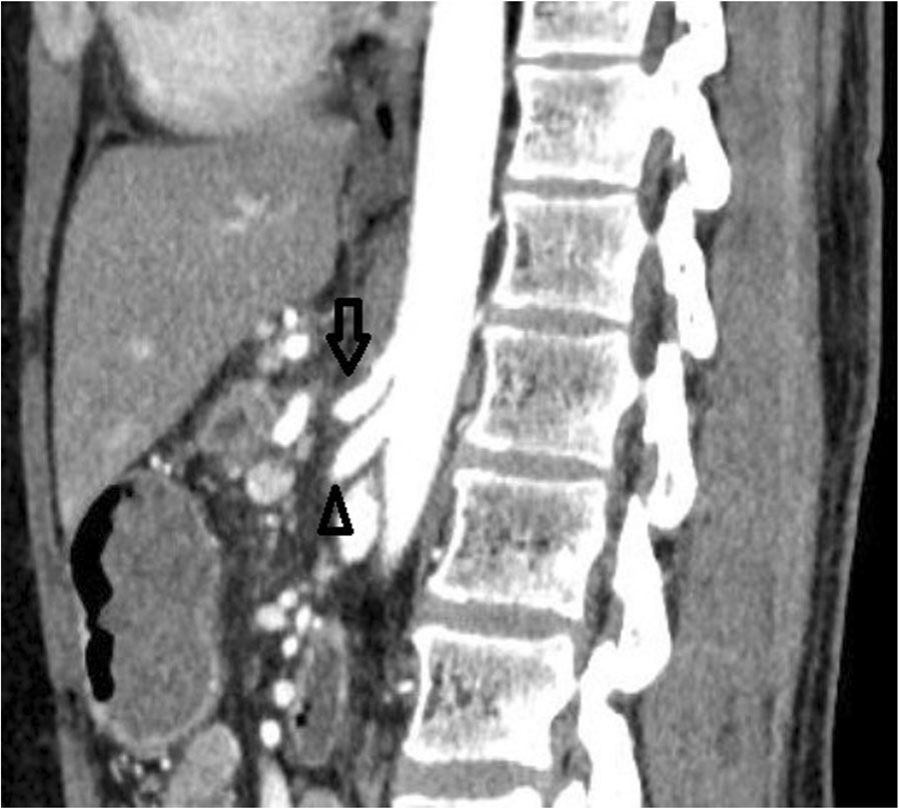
Multidetector CT with arterial reconstruction 1 day after the PD showing an acute extrinsic stenosis caused by median arcuate ligament compression
